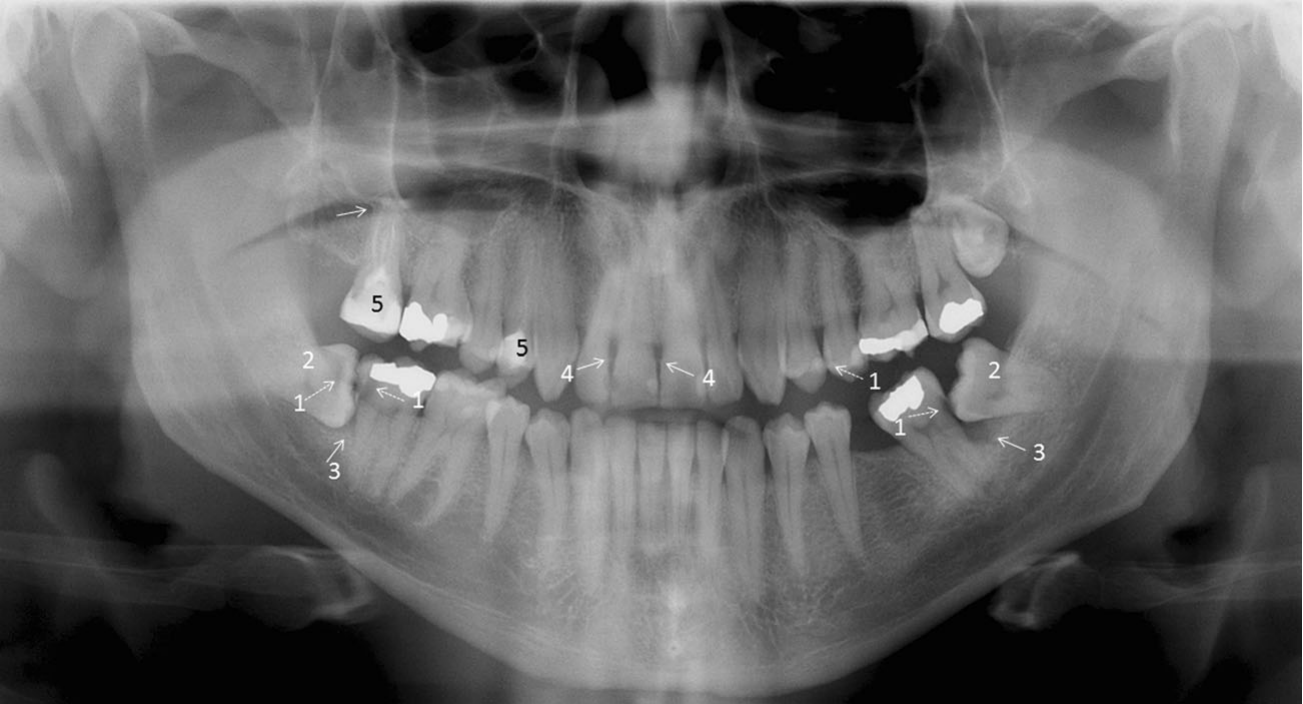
A PTG demonstrating frequent pathological conditions of teeth and periodontal (bony supportive) structures: 1. Carious lesions [dentine caries d. 37 distocervically, d. 47 distocervially, 48 occlusally and enamel caries d. 25 mesially (*dashed arrows*)]; bitewing radiography would be helpful for caries diagnostics. 2. Partially, mesioangulary erupted dd. 38, 48 and chronic pericoronitis with sclerosing osteitis next to d. 38 crown. 3. Alveolar bone loss in the region of dd. 37 and 47 distally associated with partially erupted dd. 38, 48 (*arrows*). 4. Calculus deposit best visible in dd. 12, 21 (*arrows*). 5. Dd. 17, 14 with inadequate root fillings; apical periodontitis of d. 17 (*arrow*) cannot be excluded and a periapical radiography is indicated. Also opacity of the floor of the right maxillary sinus is suspectedDuring the systematic approach of a PTG, the cortical border of the mandible must be followed and assessed for its integrity around the entire bone [15]. Also the condition of the outline of the mandibular canal should be assessed to exclude pathology [9]. It has to be remembered that only severe changes of the condylar head and glenoid fossa can be evaluated on a PTG (Fig. 8) [17]. Further investigation of the temporomandibular joint (TMJ) and evaluation of articular surfaces requires image modalities such as (CB)CT (for the evaluation of hard tissues) and magnetic resonance imaging (MRI) (for the evaluation of soft tissues) [18, 19].
